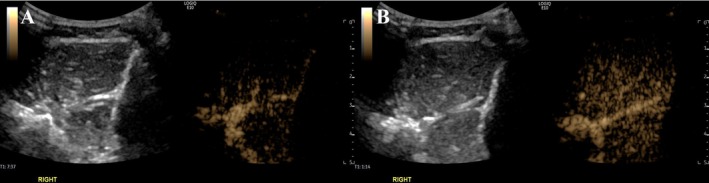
SHAPE imaging in a 28‐year‐old male CECS subject with right anterior calf compartment pain after exercise with dual grayscale ultrasound (left side of image) and subharmonic imaging (right side of image). Interestingly, in some CECS participants, there was an increase in subharmonic signal (in dB) post‐exercise, which is not fully understood at this point.
